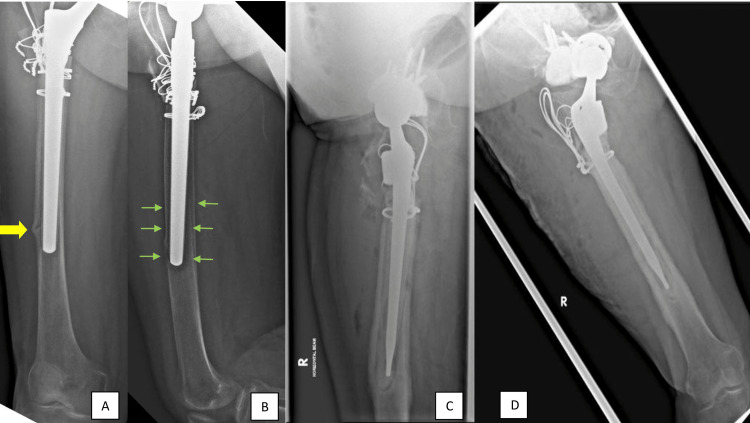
(A) Three months after the index right hip surgery, the fracture appeared to be healing with signs of callus formation (yellow arrow); (B) Two years after the surgery, plain radiograph of the right hip showed well-united fracture but also revealed peri-implant radiolucency (green arrows), suggestive of stem loosening; (C) Plain radiograph of the right hip in lateral view after the patient underwent revision surgery where a cemented stem was inserted with a revision/calcar hip system and a shortening osteotomy; (D) Plain radiograph of the right hip in anteroposterior view.
